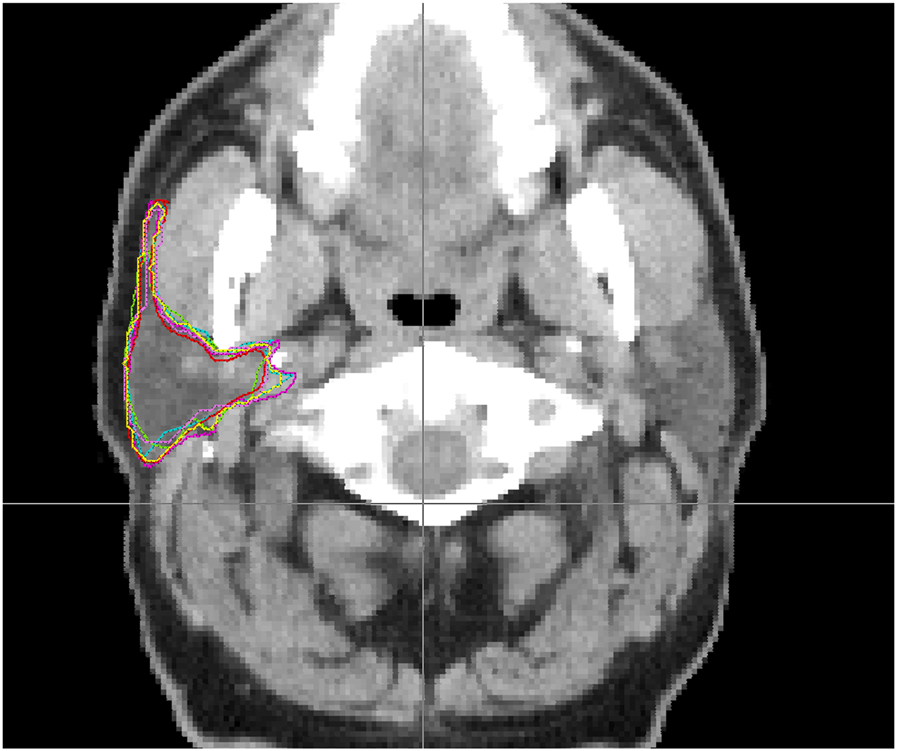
Right parotid gland defined by five manual (multiple colours) and one STAPLE (yellow) contour (one transverse CT slice shown).
